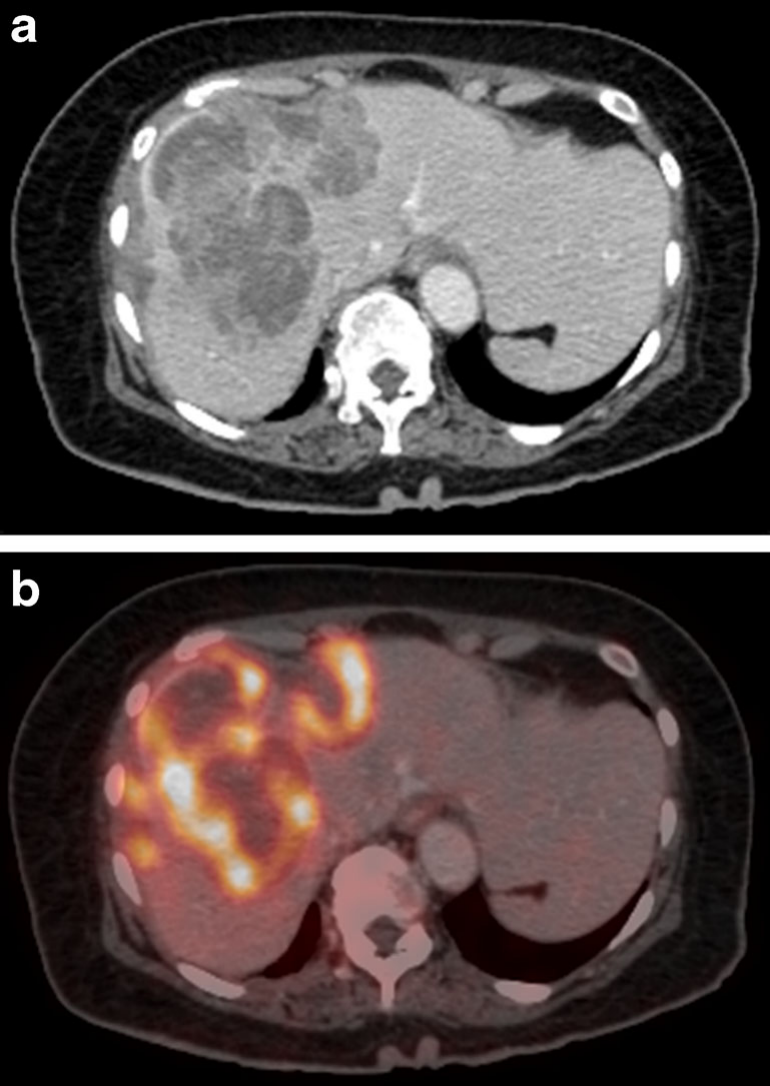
Axial contrast-enhanced CT (a) and corresponding FDG-PET (b) images of the same patient as in Fig. 6 showing avid FDG uptake within the predominantly hypoenhancing hepatic lesions (*Image courtesy: Dr. Ishita Sen, Dept. of Nuclear Medicine, Fortis Memorial Research Institute, Gurgaon*)Multifocal lesions (with or without a dominant mass)Multiple discrete hepatic lesions of varying sizes have been reported at presentation in approximately 35- 40 % of cases of PHL [10, 12], although one of the lesions is typically dominant [29]. In contrast, multifocal lesions or diffuse infiltration is the most common pattern of liver involvement in SHL, seen in up to 90 % of cases. Dominant masses are usually absent in SHL. Also, untreated nodules in SHL, even when large, are usually homogeneous (Fig. 8), while the dominant masses in PHL are typically heterogeneous. Concomitant splenic lesions can be seen in 30-40 % of patients with SHL, which, along with widespread infra- or supradiaphragmatic lymphadenopathy and bone marrow infiltration facilitate correct diagnosis [29]. The nodules are markedly hypoechoic on US. They may also have a target appearance, with central echogenic and peripheral hypoechoic components (Fig. 9). As mentioned previously, the enhancement patterns of the individual lesions in multinodular lymphoma are essentially similar to those described above for the solitary mass-forming variety of lymphoma. Thus, the nodules are typically diffusely hypoenhancing compared to the surrounding hepatic parenchyma. Patchy enhancement or rim-enhancement of the nodules can be seen in up to 15 % of cases (Figs. 10 and 11).
